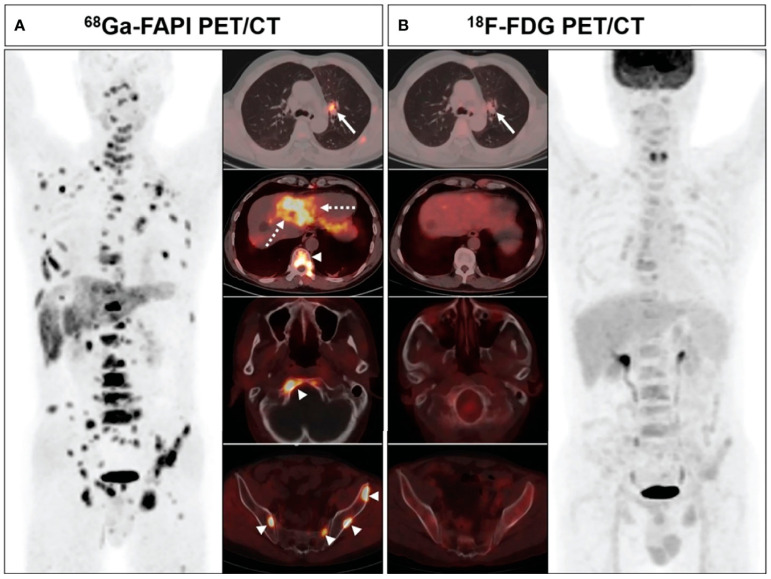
A 63-year-old male diagnosed with lung adenocarcinoma. ^68^Ga-FAPI PET/CT (A) showed intense tracer uptake in the primary tumor (solid arrows, SUVmax = 10.0), liver metastasis (dashed arrows, SUVmax = 7.6) and bone metastases (arrows, SUVmax = 8.3–8.5). FDG PET/CT (B) showed primary lesion with mild FDG uptake (solid arrows, SUVmax = 3.6), while no significant FDG uptake was showed in liver metastasis and multiple bone metastases (Reprinted/adapted with permission from Ref. [109]. Copyright © 2022 Wu, Deng, Zhong, Wang, Rao, Wang, Chen and Zhang).
